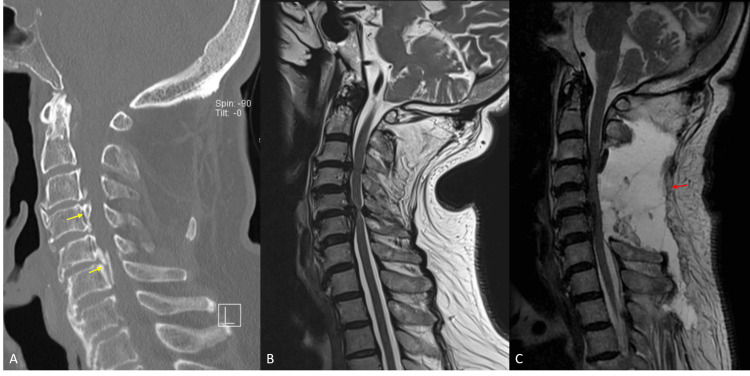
(A) Preoperative CT showing segmental OPLL (yellow arrows). (B) Preoperative MRI showing multilevel cord compression. (C) MRI on postoperative day six demonstrating compressive seroma (red arrow). OPLL: Ossification of the posterior longitudinal ligament.
